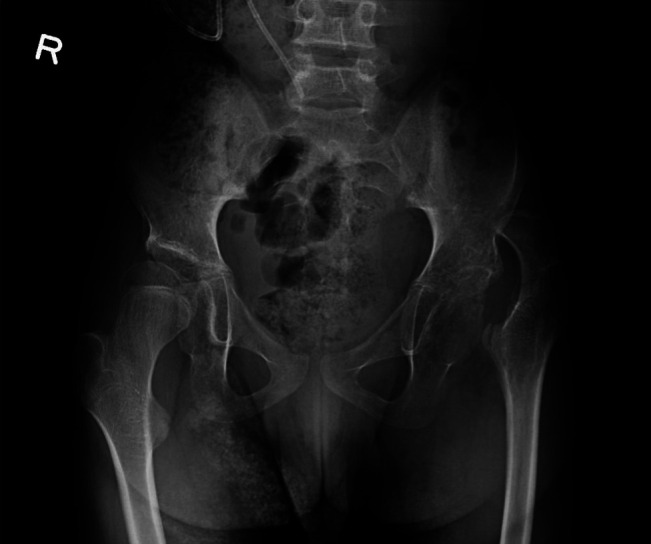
One-year postoperative anterior-posterior radiograph after two irrigation and débridement surgeries of left hip joint for septic hip arthritis revealing a high riding left hip dislocation.
